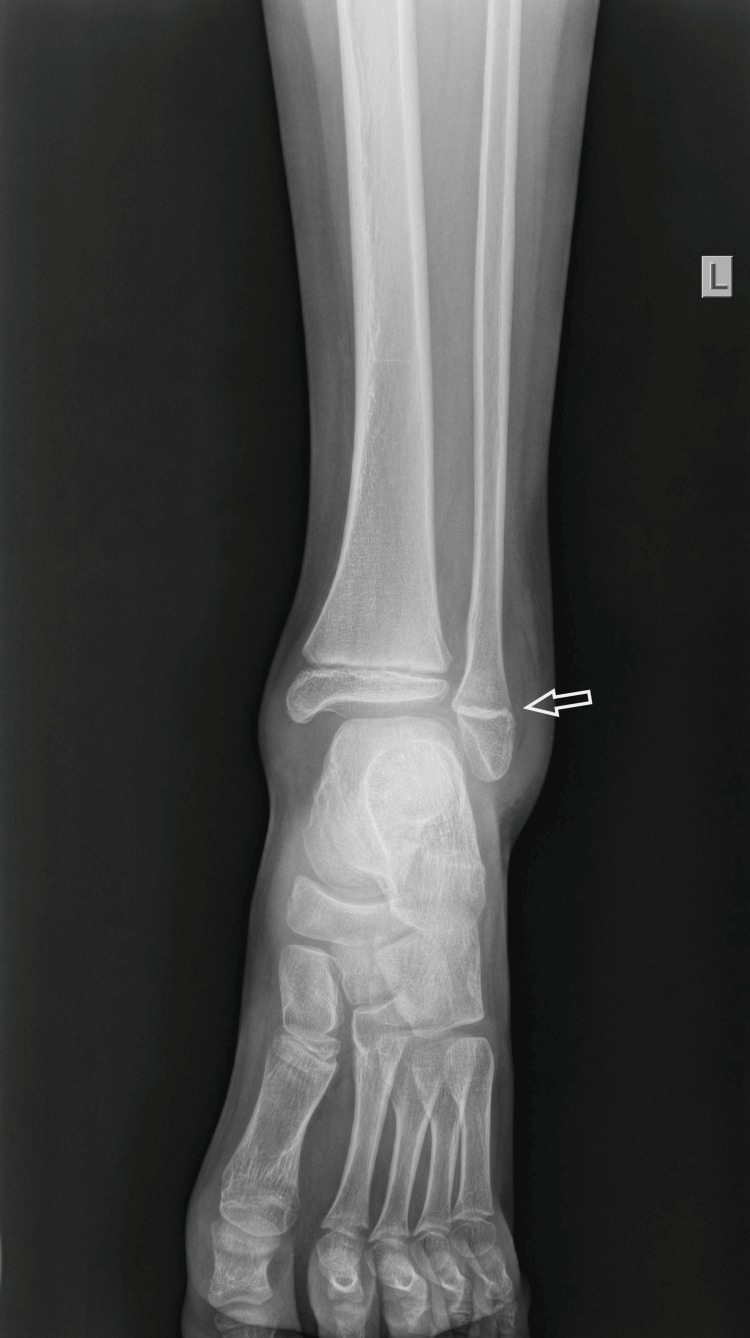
Reviewing the initial AP X-ray of the ankle joint, with the arrow pointing to the reduced width of the distal physis of the fibula.
